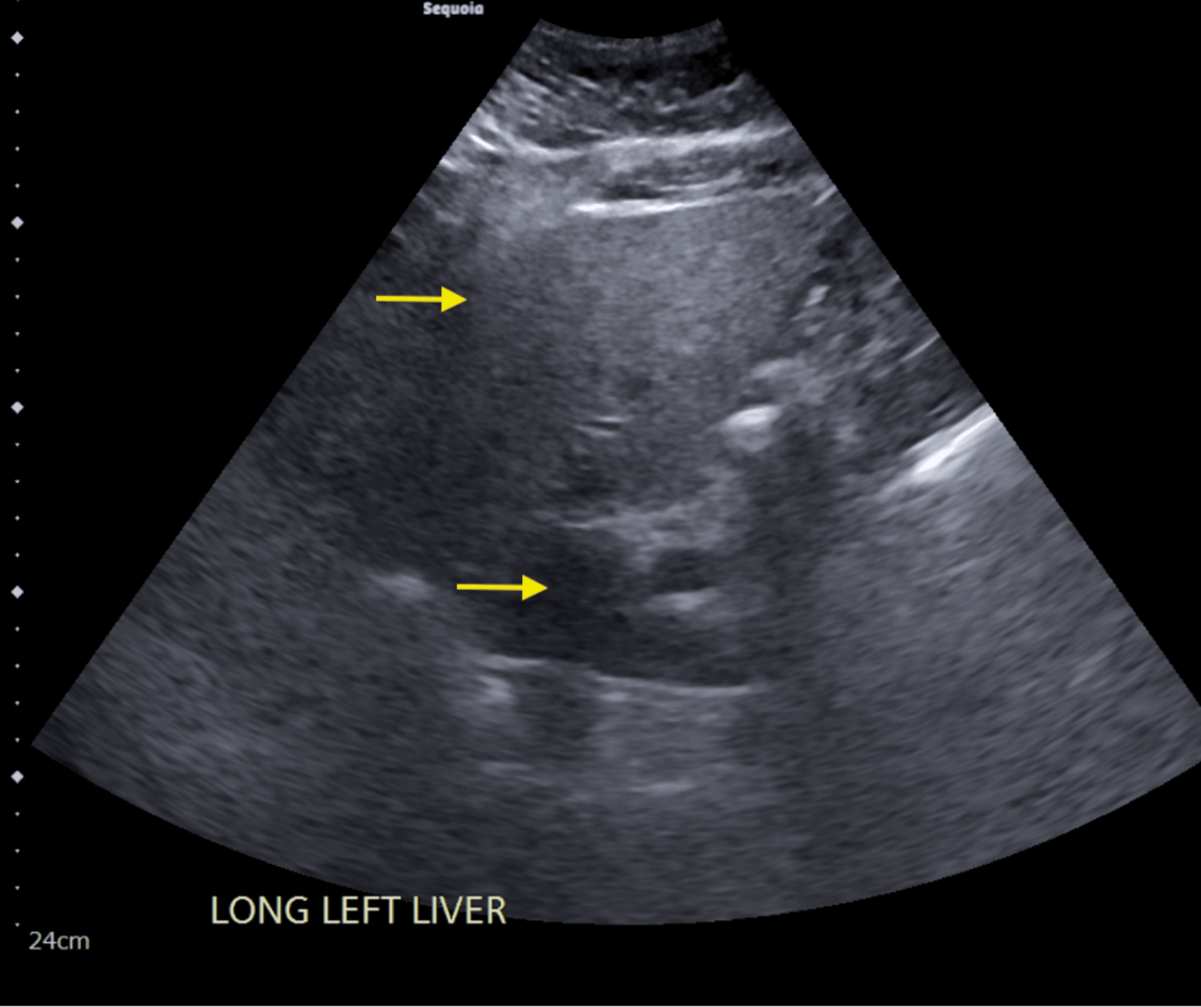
Ultrasound of liver showing mild hepatomegaly and hepatic steatosis
